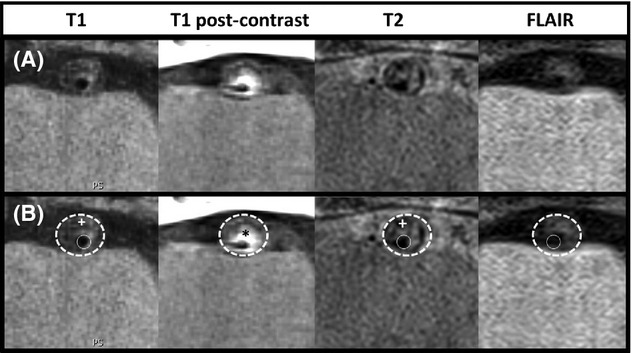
HRMRI of basilar atherosclerosis at level of the stenosis. Top row (A) T1 pre- and postcontrast, T2, and FLAIR images. Bottom row (B) shows same images with white dashed circle outlining artery and thin white circle outlining lumen. Lipid (white +) is isointense on T1 and hypointense on T2. Contrast enhancement of plaque (*) demonstrates ruptured fibrous cap.
